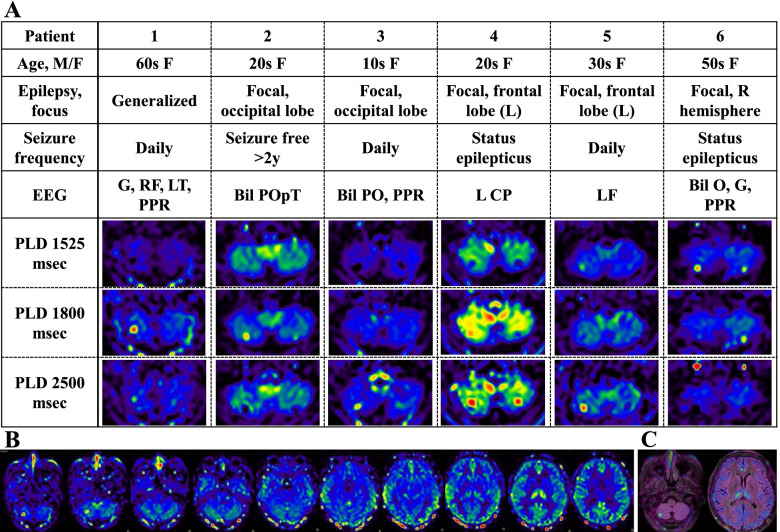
A Six patients with epilepsy, showing hyperperfusion of ASL in selective areas the cerebellar paravermis of lobule VIIb. Four patients (Patient 1, 3, 4, and 5) showed hypoperfusion of the VIIb lobule in short PLD conditions (1525 msec in Patient 1 and Patient 4, 1525 and 1800 msec in Patient 3, and 1800 msec in Patient 5) and showed hyperperfusion of the same region in longer PLD conditions. In Patient 4 and Patient 5 with focal epilepsy manifesting with asymmetrical motor symptoms, cerebellar hyperperfusion was found on the opposite side to the seizure focus estimated by seizure semiology. B Axial ASL images of Patient 1. At the bottom of the right cerebellum, hypoperfusion in PLD 1525 msec and hyperperfusion in PLD 1800 and 2500 msec are seen. In the left thalamus, hypoperfusion in PLD 1800 and 2500 msec is shown. C Co-registration of ASL to FLAIR images. ASL: arterial spin labeling, PLD: post labeling delay, FLAIR: fluid-attenuated inversion-recovery, M/F: male/female, EEG: electroencephalogram, R: right, L: left, Bil: bilateral, G: generalized, F: frontal, C: central, P: parietal, O: occipital, T: temporal, pT: posteriotemporal, PPR: photoparoxysmal response
